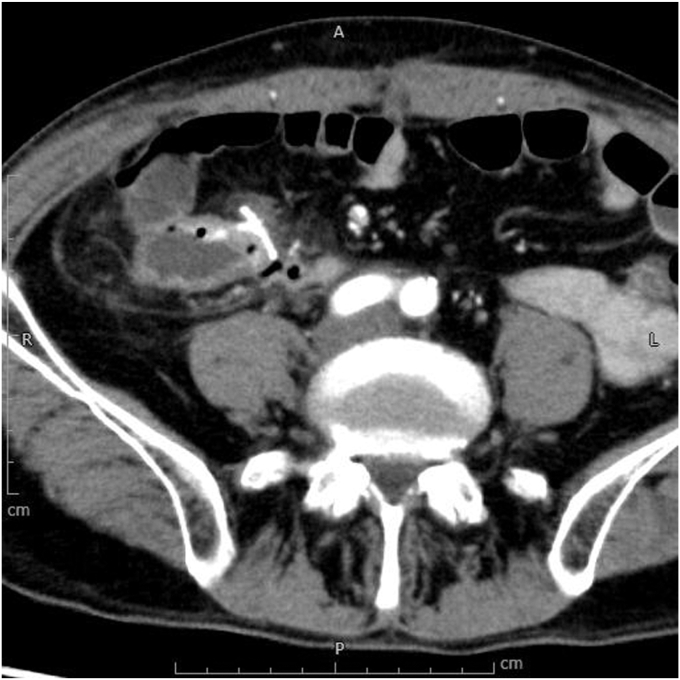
Urgent contrast-enhanced CT scan demonstrating mucosal enhancement on both sides of the ileocolonic anastomosis, along with thickening of the surrounding adipose tissue.
